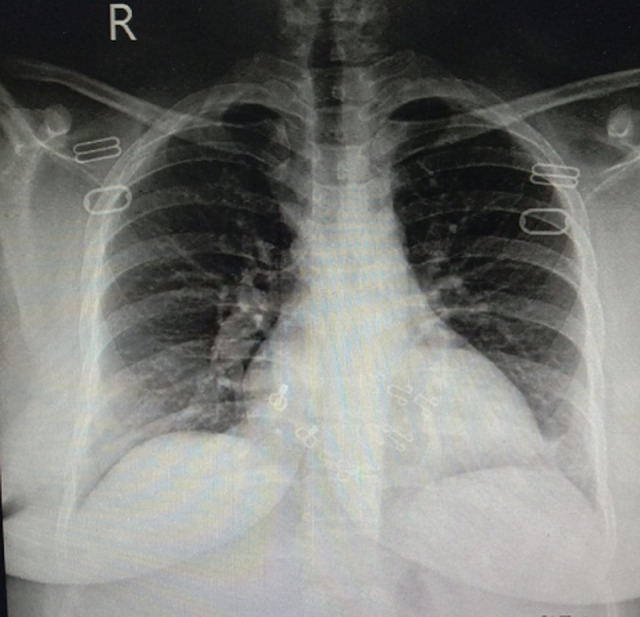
chest X-ray (AP view) showing perihilar prominence with bilateral interstitial infiltrate
